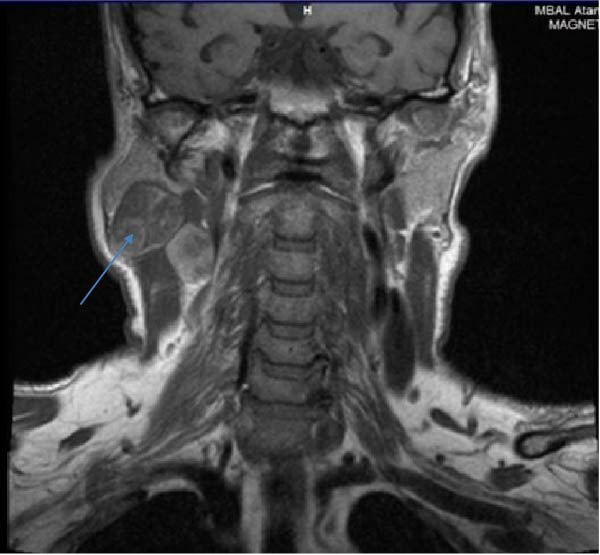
Magnetic resonance imaging showing a right parotid gland mass.
